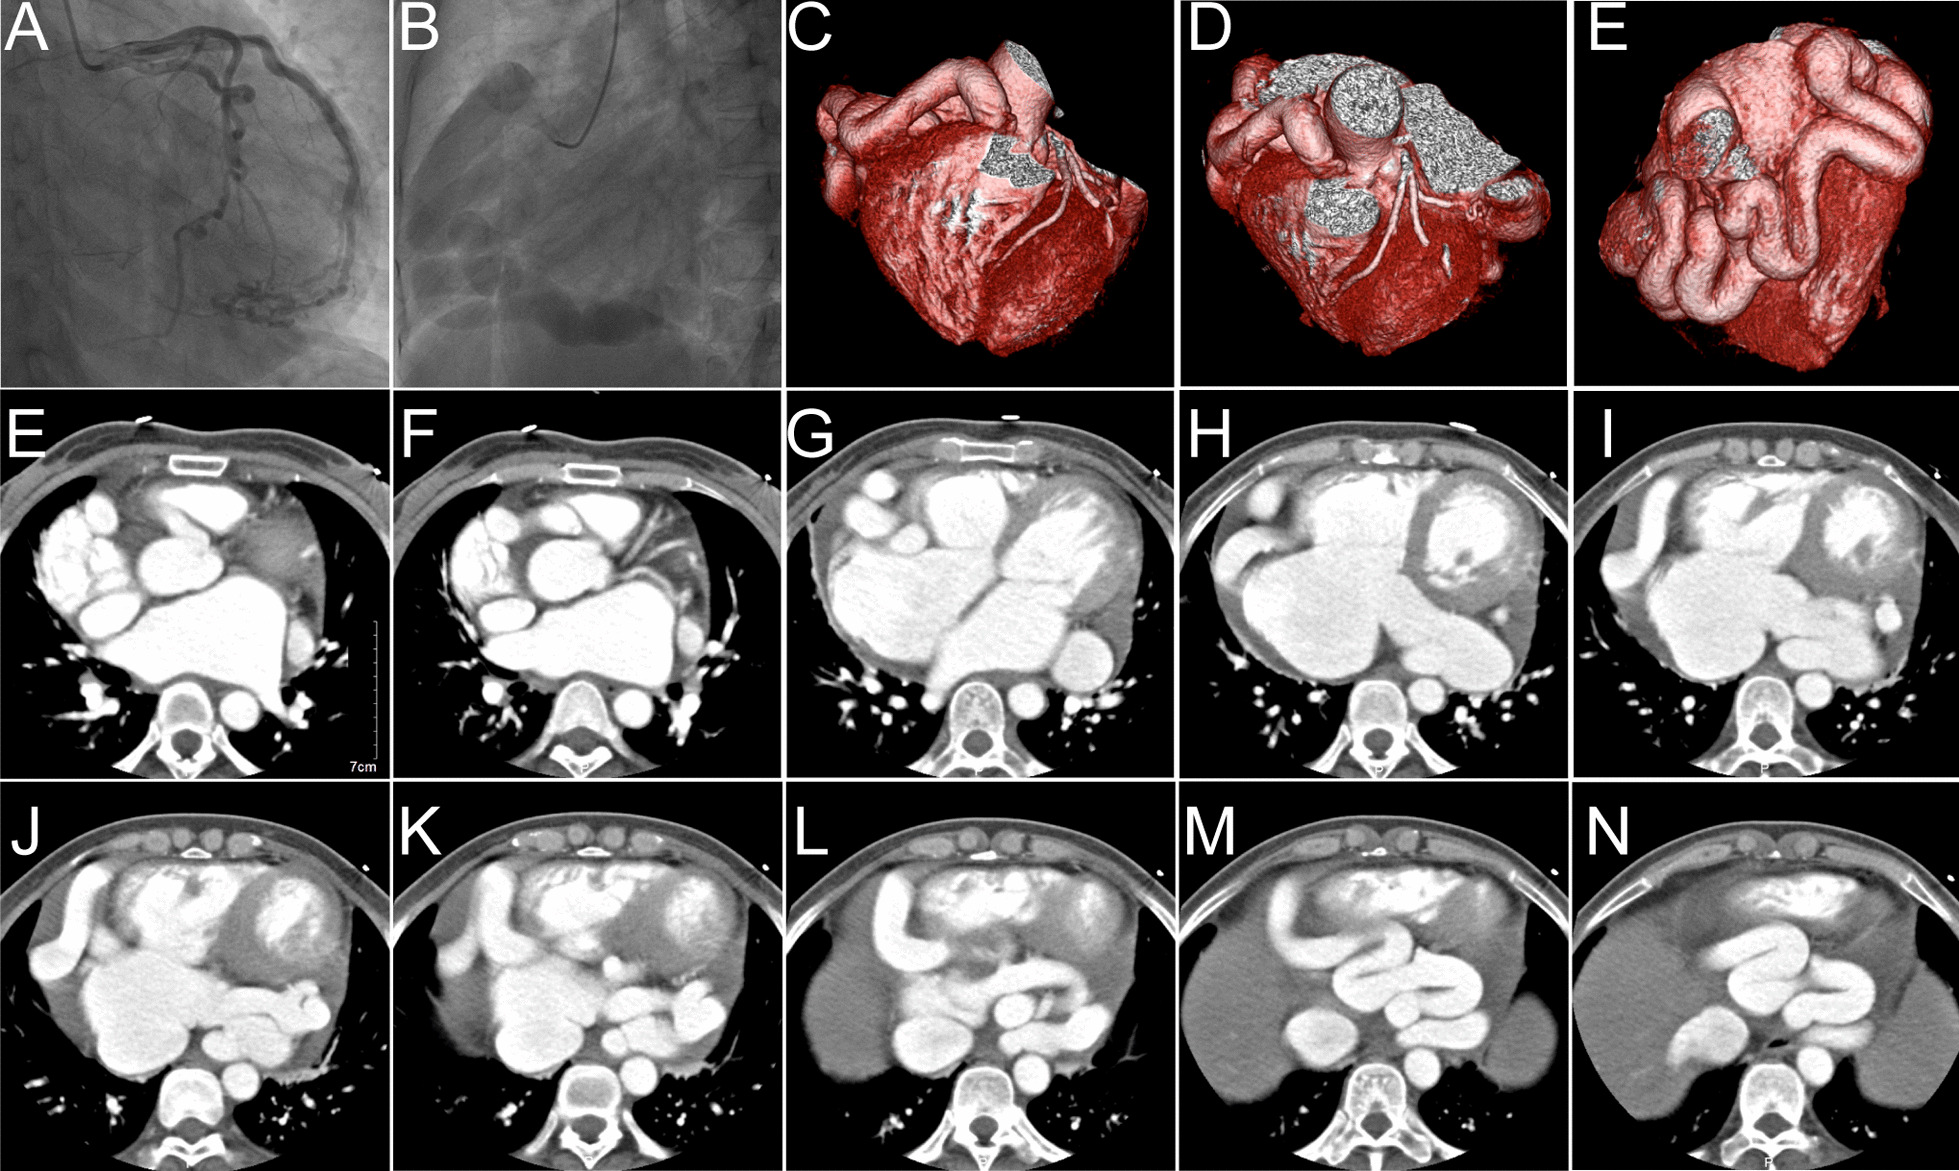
A, B Coronary angiography demonstrated normal left coronary artery blood flow and tortuously dilated right coronary artery. C–N Preoperative computed tomography angiography revealed that the whole course of RCA was severely dilated, tortuous RCA and abnormally fistulized to the posterior and inferior aspects of coronary sinus. The widest dimension of RCA was about 2 cm. Imaging findings also indicated the presence of persistent left superior vena cava (LPSV)
